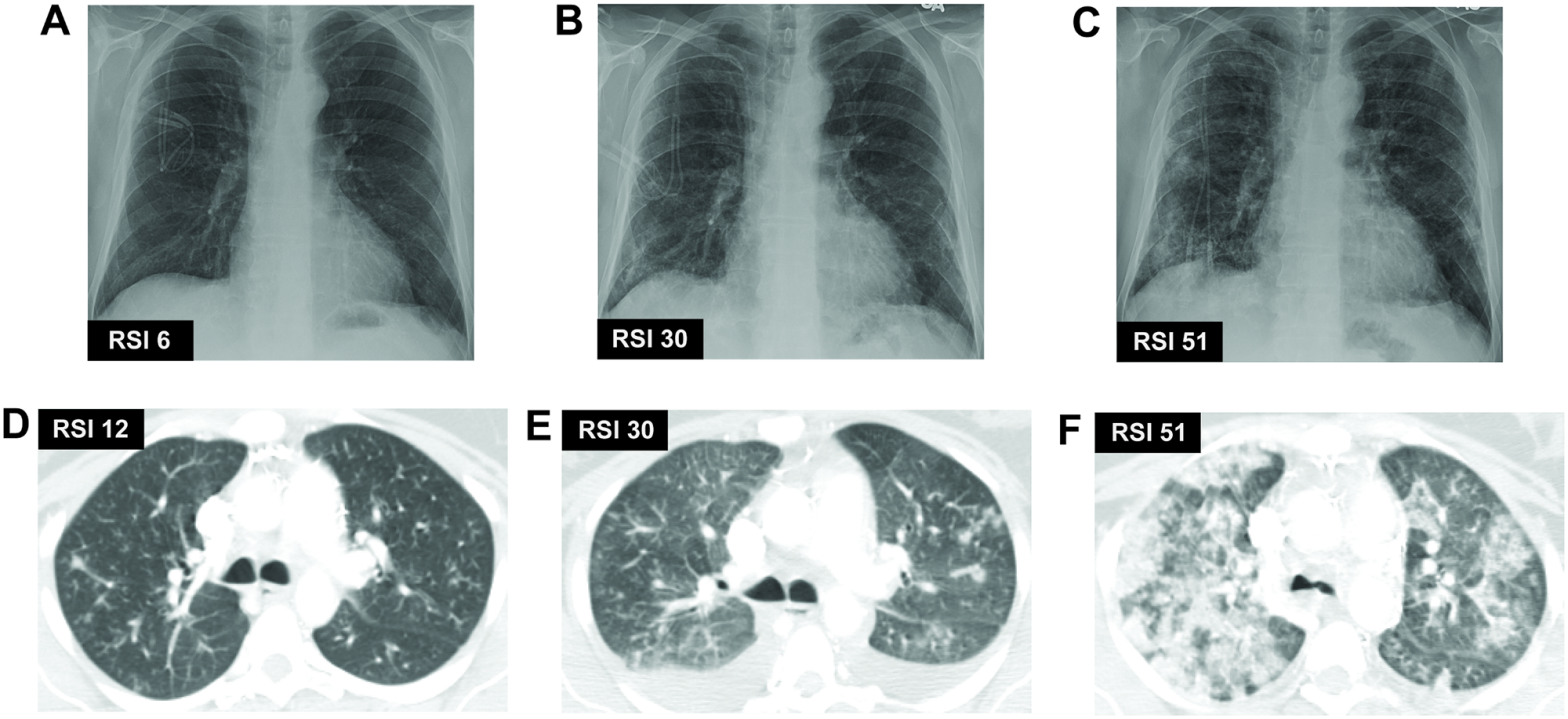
Representative images of RSI scoring for CXR and CT images. RSI scores are labelled within each panel. Panels (A)-(C) show CXR images from an individual patient in order of increasing severity. Panels (D)-(F) show CT images from a different individual patient in order of increasing severity.
